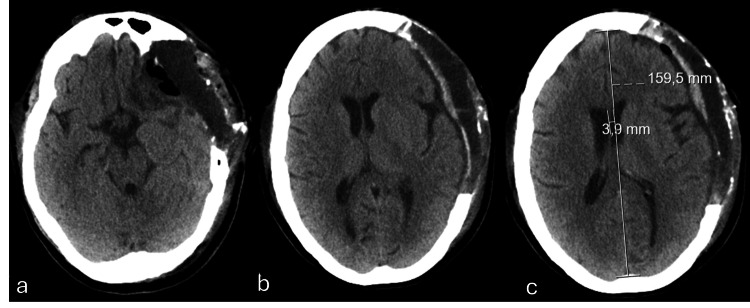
Axial plane of brain computed tomography obtained after adopting measures opposing intracranial hypotension There is a marked reduction in the existing anterior cerebral edema and consequent mass effect (a), with greater patency of the left hemispheric sulci and the lateral ventricle of the same side (b), persisting only with an incipient deviation of the structures from the midline, estimated at around 4mm to the right (c).
